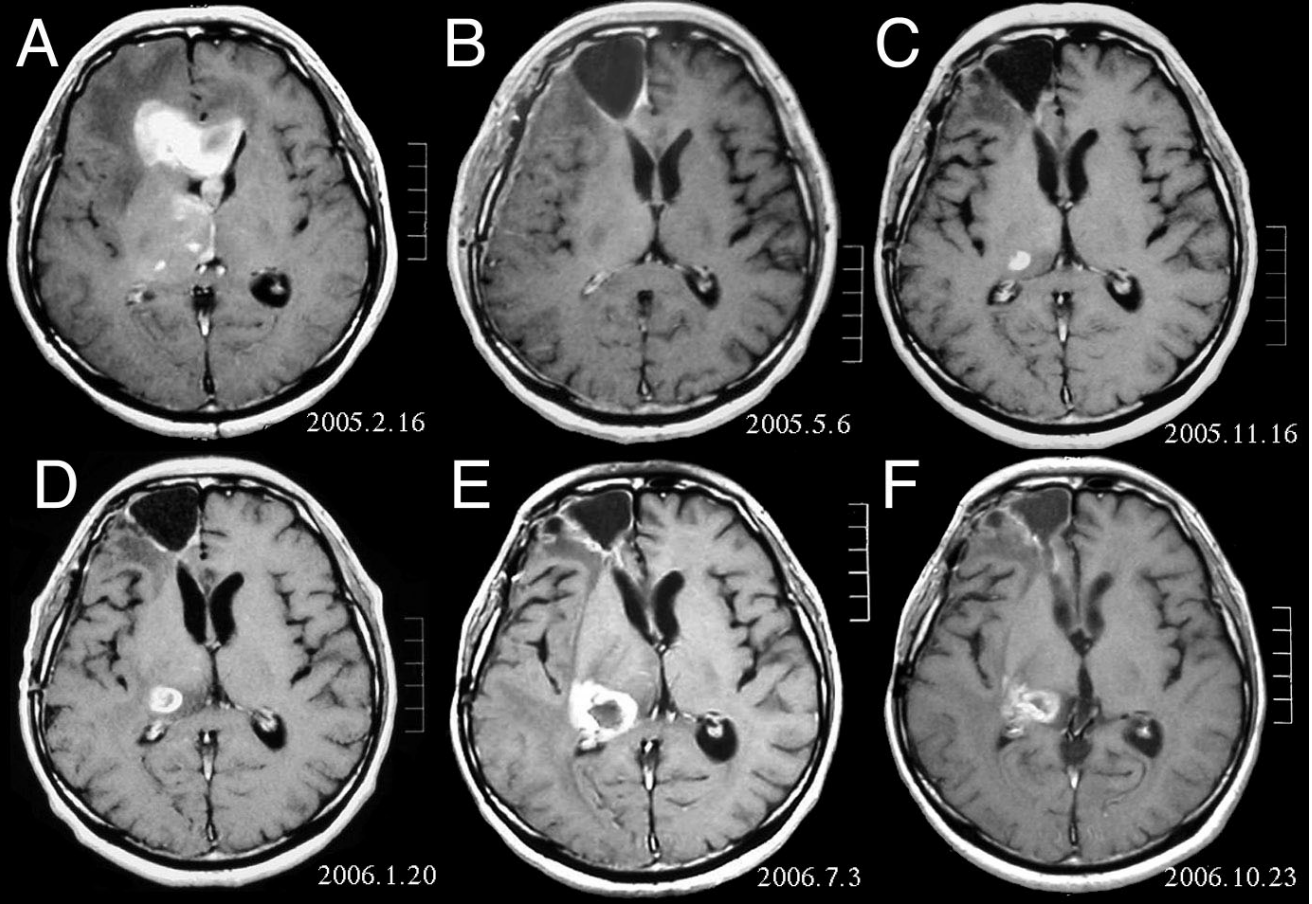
MRI of brain. (A) Initial MRI on February 16, 2005, shows a tumor in the right and left frontal lobe as well as the right thalamus. (B) MRI after surgery, radiation and chemotherapy. The tumor has completely disappeared except for slight enhancement adjacent to the surgical margin. (C) Recurrence of the thalamic tumor despite maintenance chemotherapy on November 16, 2005. (D) Increase in size of the thalamic tumor two months after stereotactic radiotherapy. (E) After 6 cycles of TMZ therapy, the thalamic lesion enlarged, and the patient developed dysarthria and hemiparesis. (F) After 2 courses of treatment with interferon-beta and TMZ, the tumor shows a partial response.
